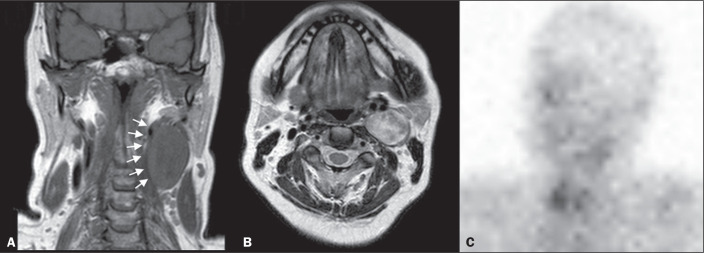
MRI scan of patient A2, in whom the SRS result was positive and the final
